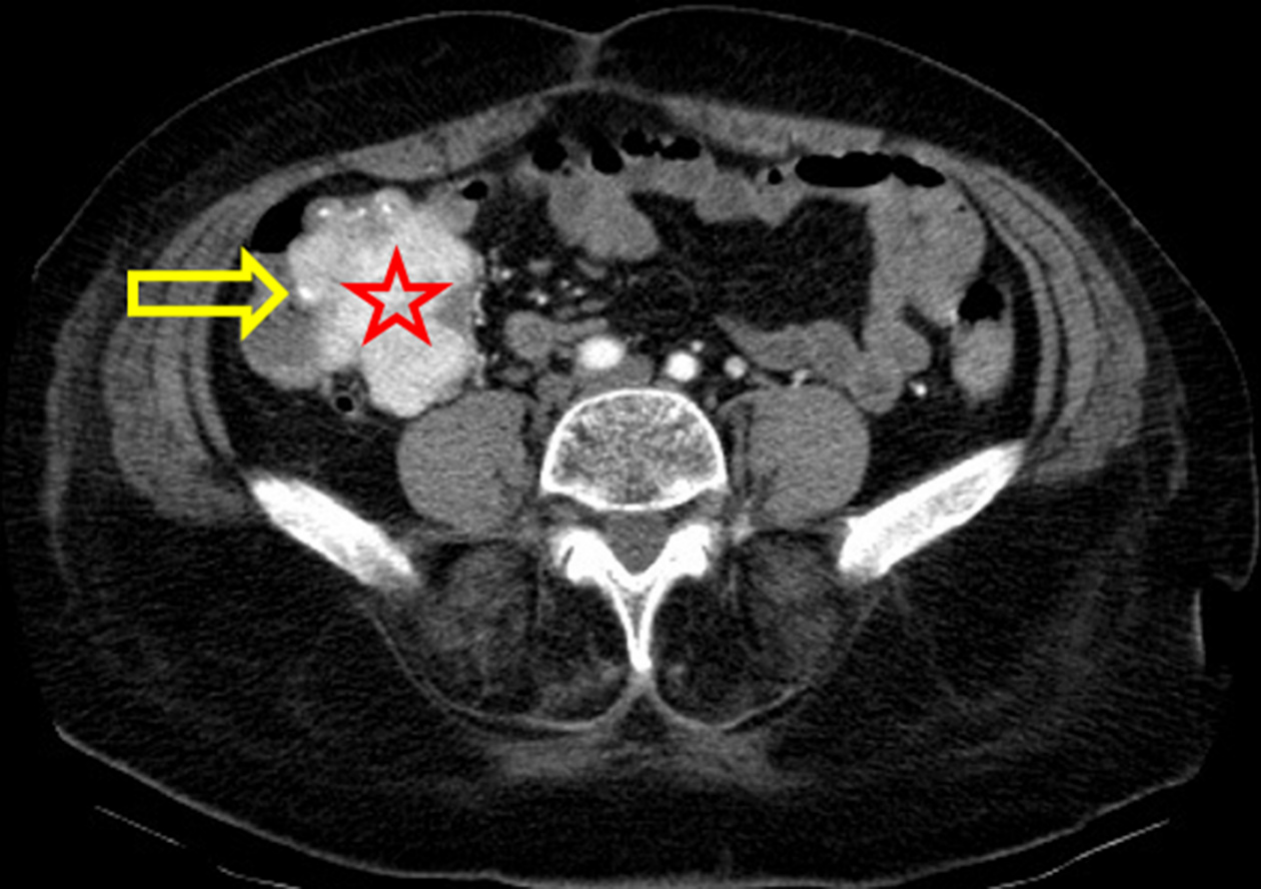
Axial enhanced CT scan, arterial phase. Heterogeneous enhancing mass (red star) in the wall of terminal ileum. Small jet of active contrast extravasation in the lumen of the cecum (yellow arrow).
